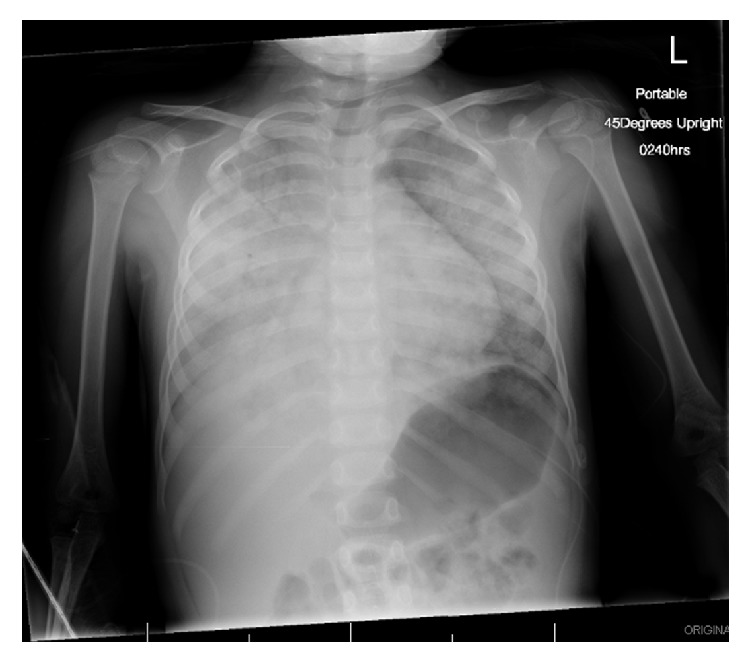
Radiograph on day of admission. Radiograph showing dense consolidation of the right lung obscuring the cardiothymic silhouette and tracheal deviation towards the right. Bilateral consolidation with associated air bronchograms.
